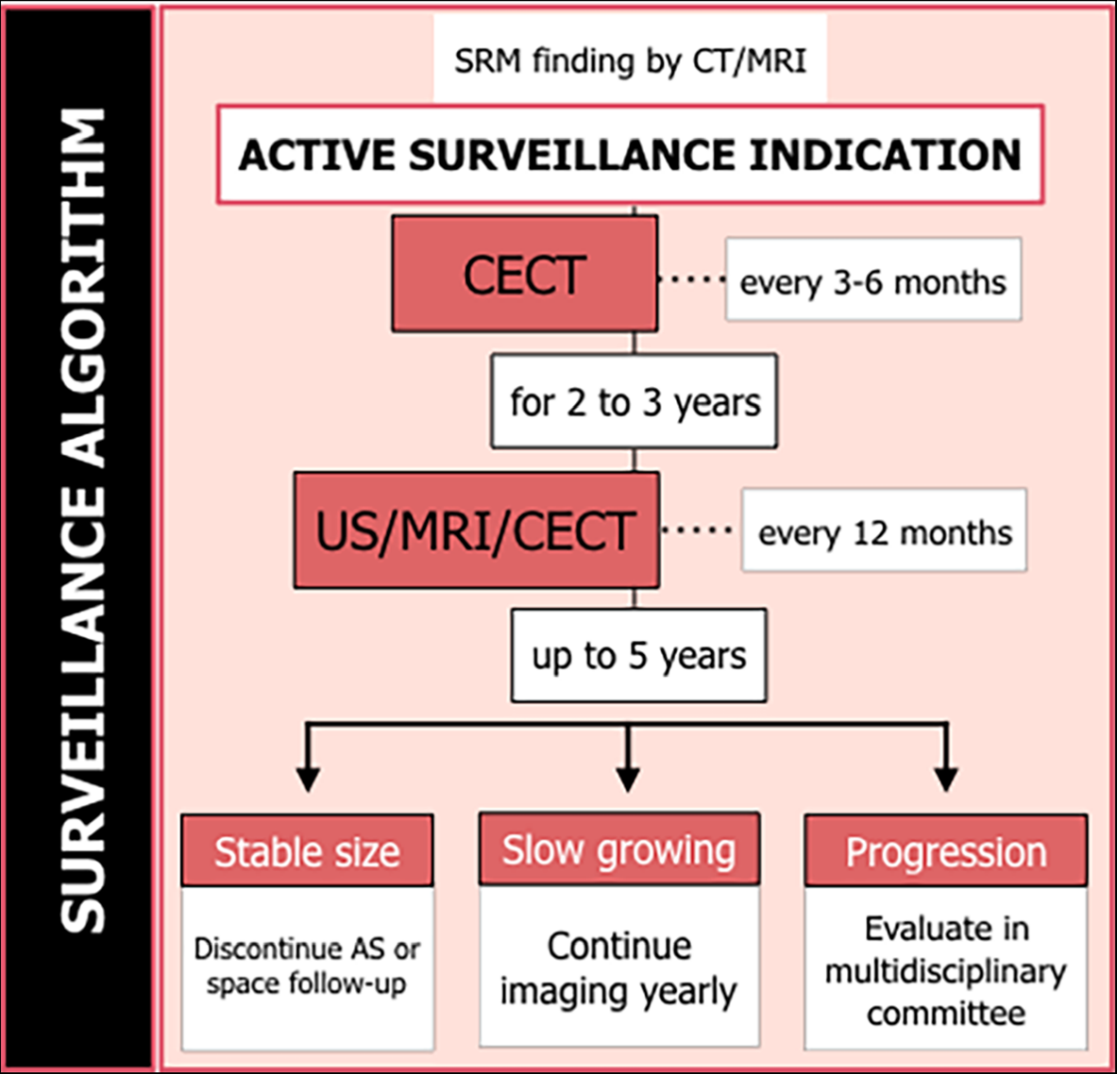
Suggested active surveillance management algorithm. Contrast-enhanced computerized tomography (CECT), ultrasound (US), and magnetic resonance (MR)
